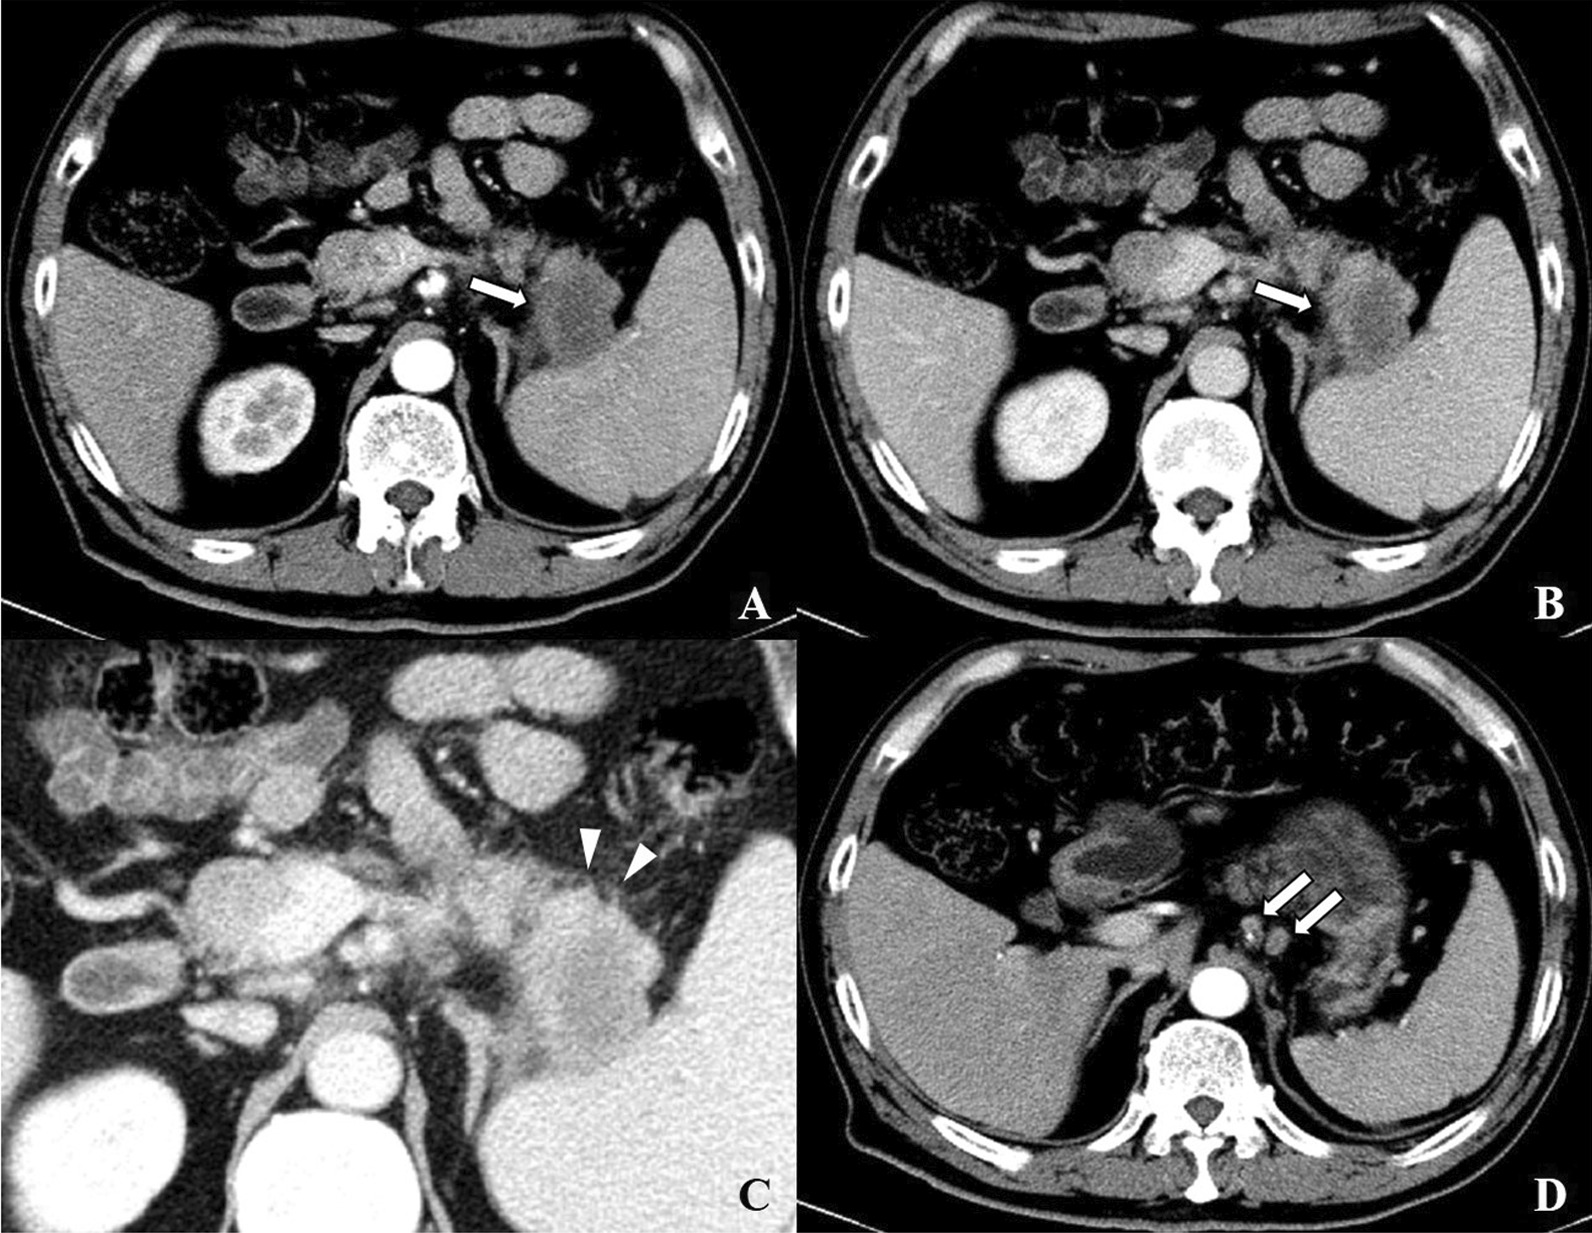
A 62-year-old man with elevated CA19-9. Preoperative CT detected a solid lesion in pancreatic tail. Moderately differentiated pancreatic ductal adenocarcinoma was confirmed after extended distal pancreatectomy. Contrast-enhanced CT images demonstrate a 4.6-cm hypodense mass in pancreatic tail (arrow) (C) in (A) arterial phase and (B) portal venous phase. Peripancreatic infiltration is appeared(arrowhead). Two enhanced suspicious metastatic lymph nodes (D) are observed (arrow). NLR is 2.96. Tumor recurrence occurrence 5.6 months and died 15.1 months after surgery
